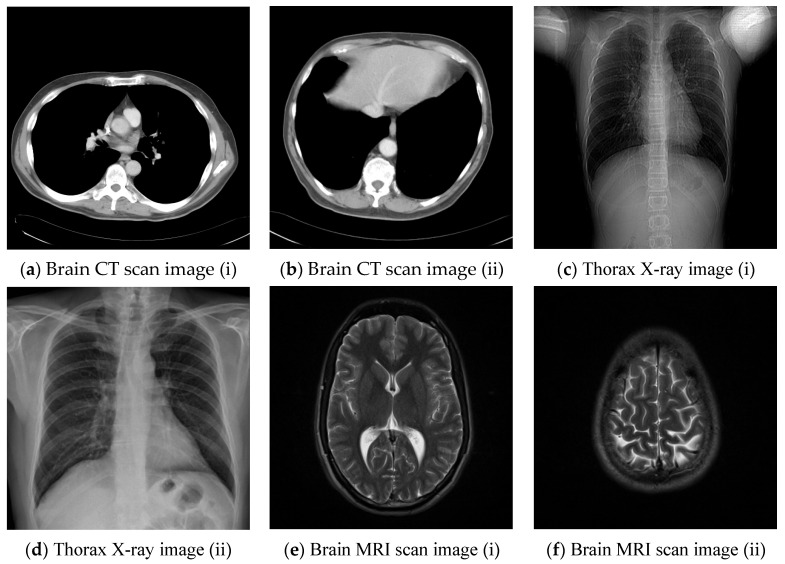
Six medical test images; (a) Brain CT scan image (i); (b) Brain CT scan image (ii); (c) Thorax X-ray image (i); (d) Thorax X-ray image (ii); (e) Brain MRI scan image (i); (f) Brain MRI scan image (ii).
